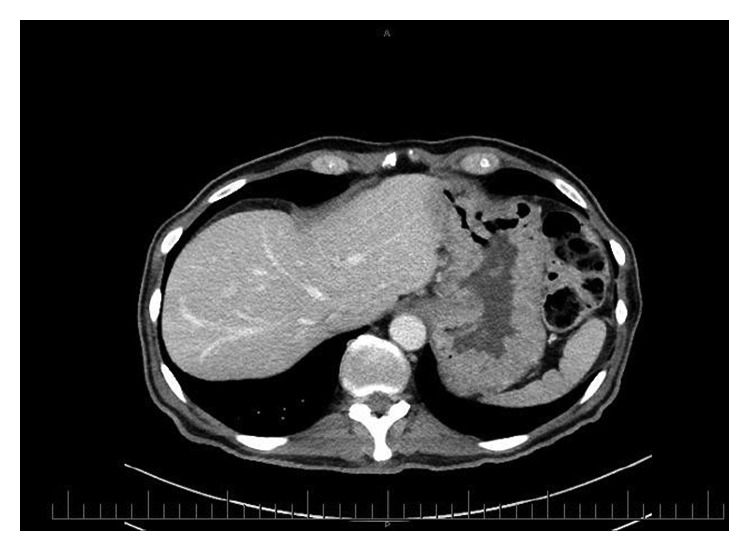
Abdominal CT on admission demonstrating hypertrophic gastric folds.
